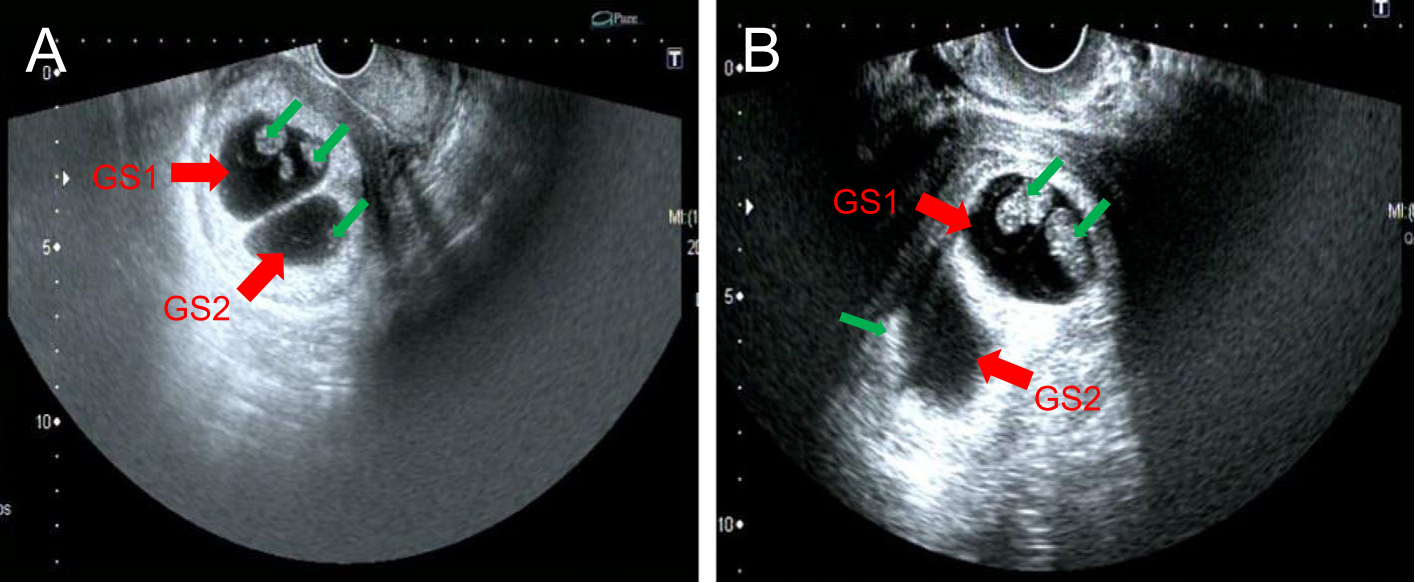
Dichorionic triplets were diagnosed by transvaginal ultrasound, two gestational sacs (GSs, red arrowhead) and three germs (green arrowhead) were detected inside the uterus. A) Ultrasound image of first pregnancy at 5 weeks post-transfer. B) Ultrasound image of second pregnancy on day 37 post-transfer
